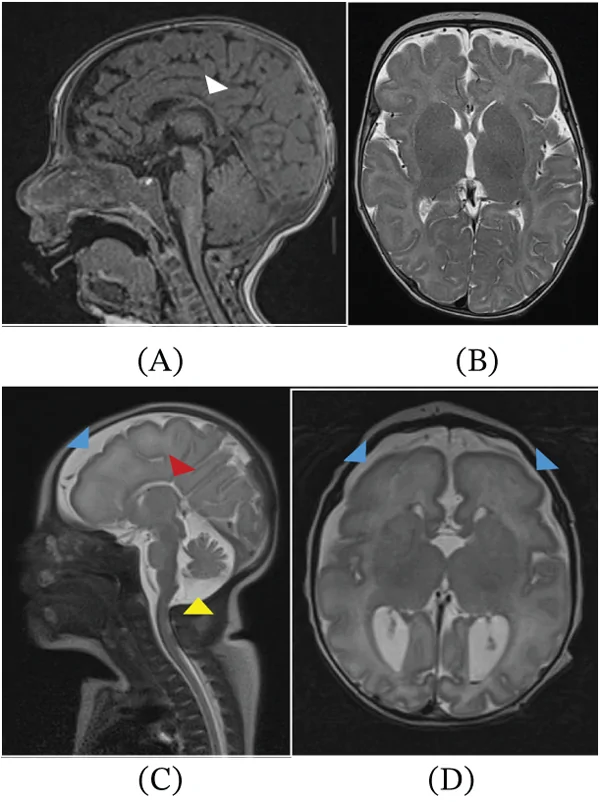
Brain magnetic resonance imaging (MRI) findings in the two patients with *KAT6B*‐related disorders. Patient 1: (A) sagittal T1‐weighted and (B) axial T2‐weighted images demonstrating thinning of the corpus callosum (white arrow). Patient 2: (C) sagittal and (D) axial T2‐weighted images showing complete agenesis of the corpus callosum (red arrow), marked gyral simplification involving the frontal and anterior temporal lobes (light blue arrows), and cerebellar vermian hypoplasia (yellow arrow).
